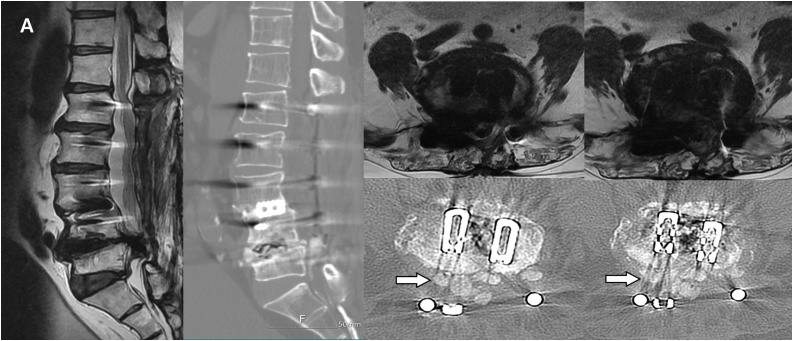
A. T2-weighted magnetic resonance imaging shows low-signal material at the L4-5 level. B. Three-dimensional computed tomography in the sagittal view shows local kyphosis and L5 body collapse, with a bone-like lesion at the L4-5 level. C, D. Calcified material protruded to the intracanal area and compressed the cauda equina fiber.
